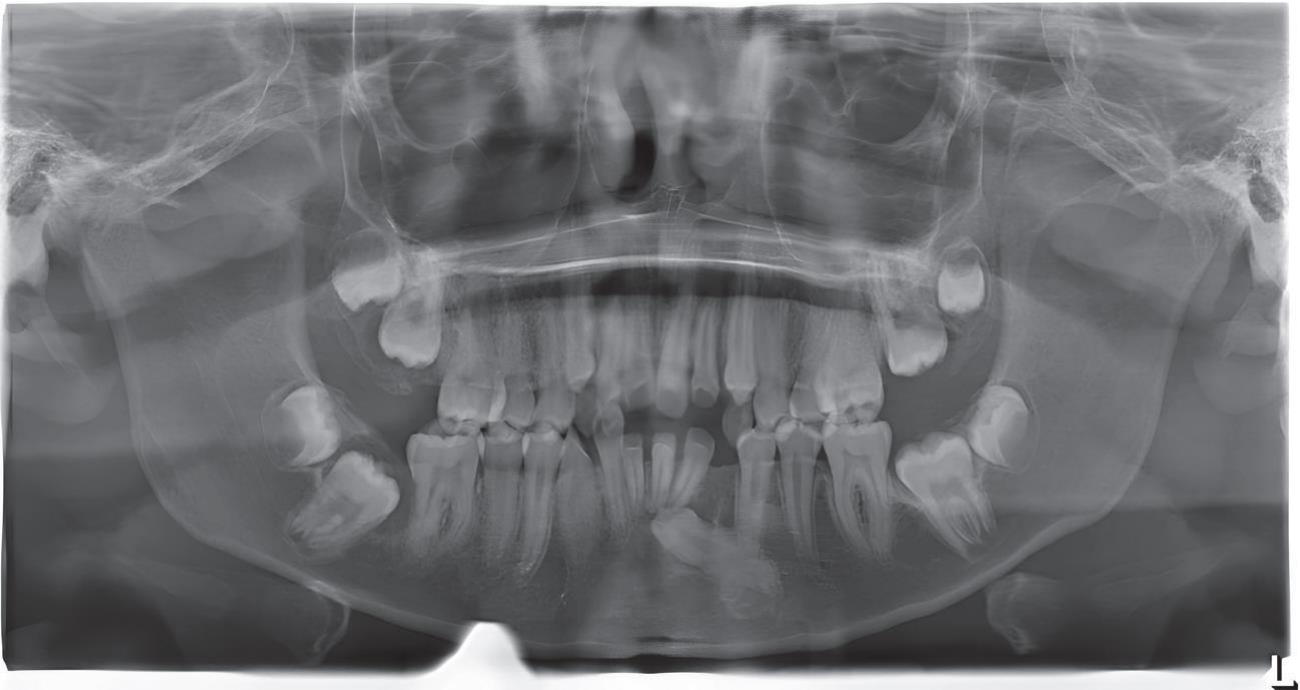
Panoramic radiograph of the 14‐year‐old patient with Ask‐Upmark kidney showing the teeth present. Some teeth show eruption deviation and a great tendency to impaction.
